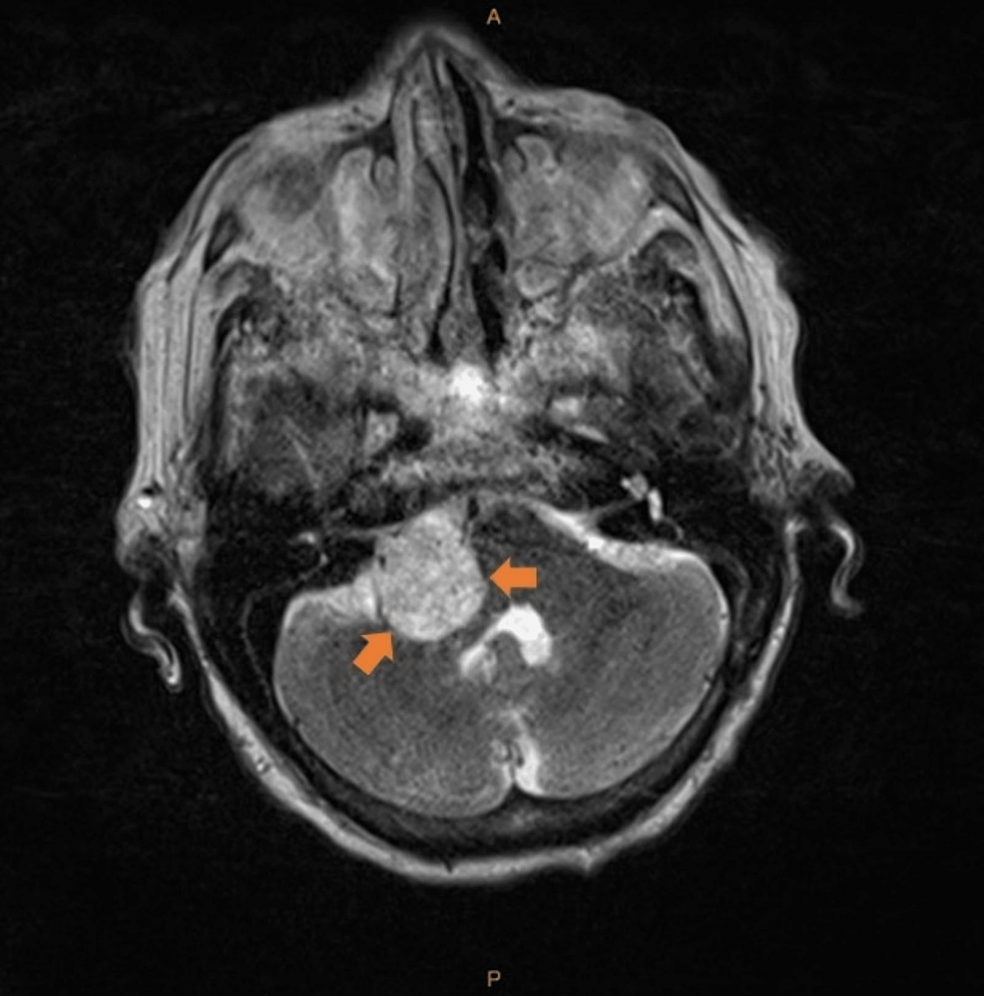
Axial T2 turbo spin-echo (TSE) magnetic resonance imaging (MRI) brain showing right cerebellopontine angle mass. A- anterior, P- posterior.
